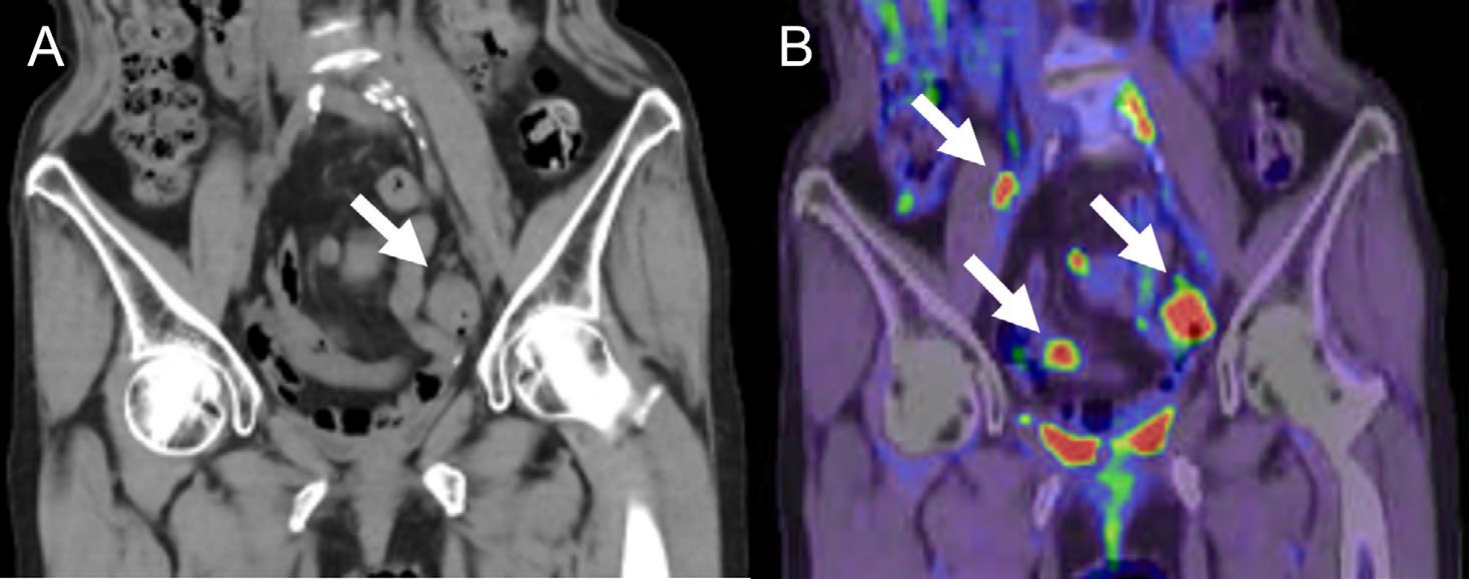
Radiographic findings after the second video‐assisted thoracic surgery. (A) A plain, non‐contrast pelvic computed tomography (CT) demonstrated an enlarged left common iliac lymph node swelling, while (B) fluorodeoxyglucose positron emission tomography/CT revealed multiple hot spots in the pelvic area.
